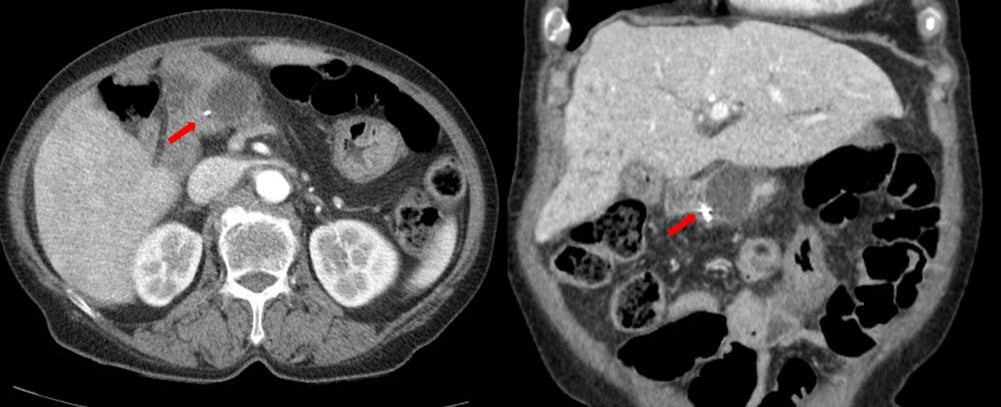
Abdominal computed tomography showed the increased size (3.5 cm) of the pancreatic pseudocyst and an endoclip (red arrow) next to it.
